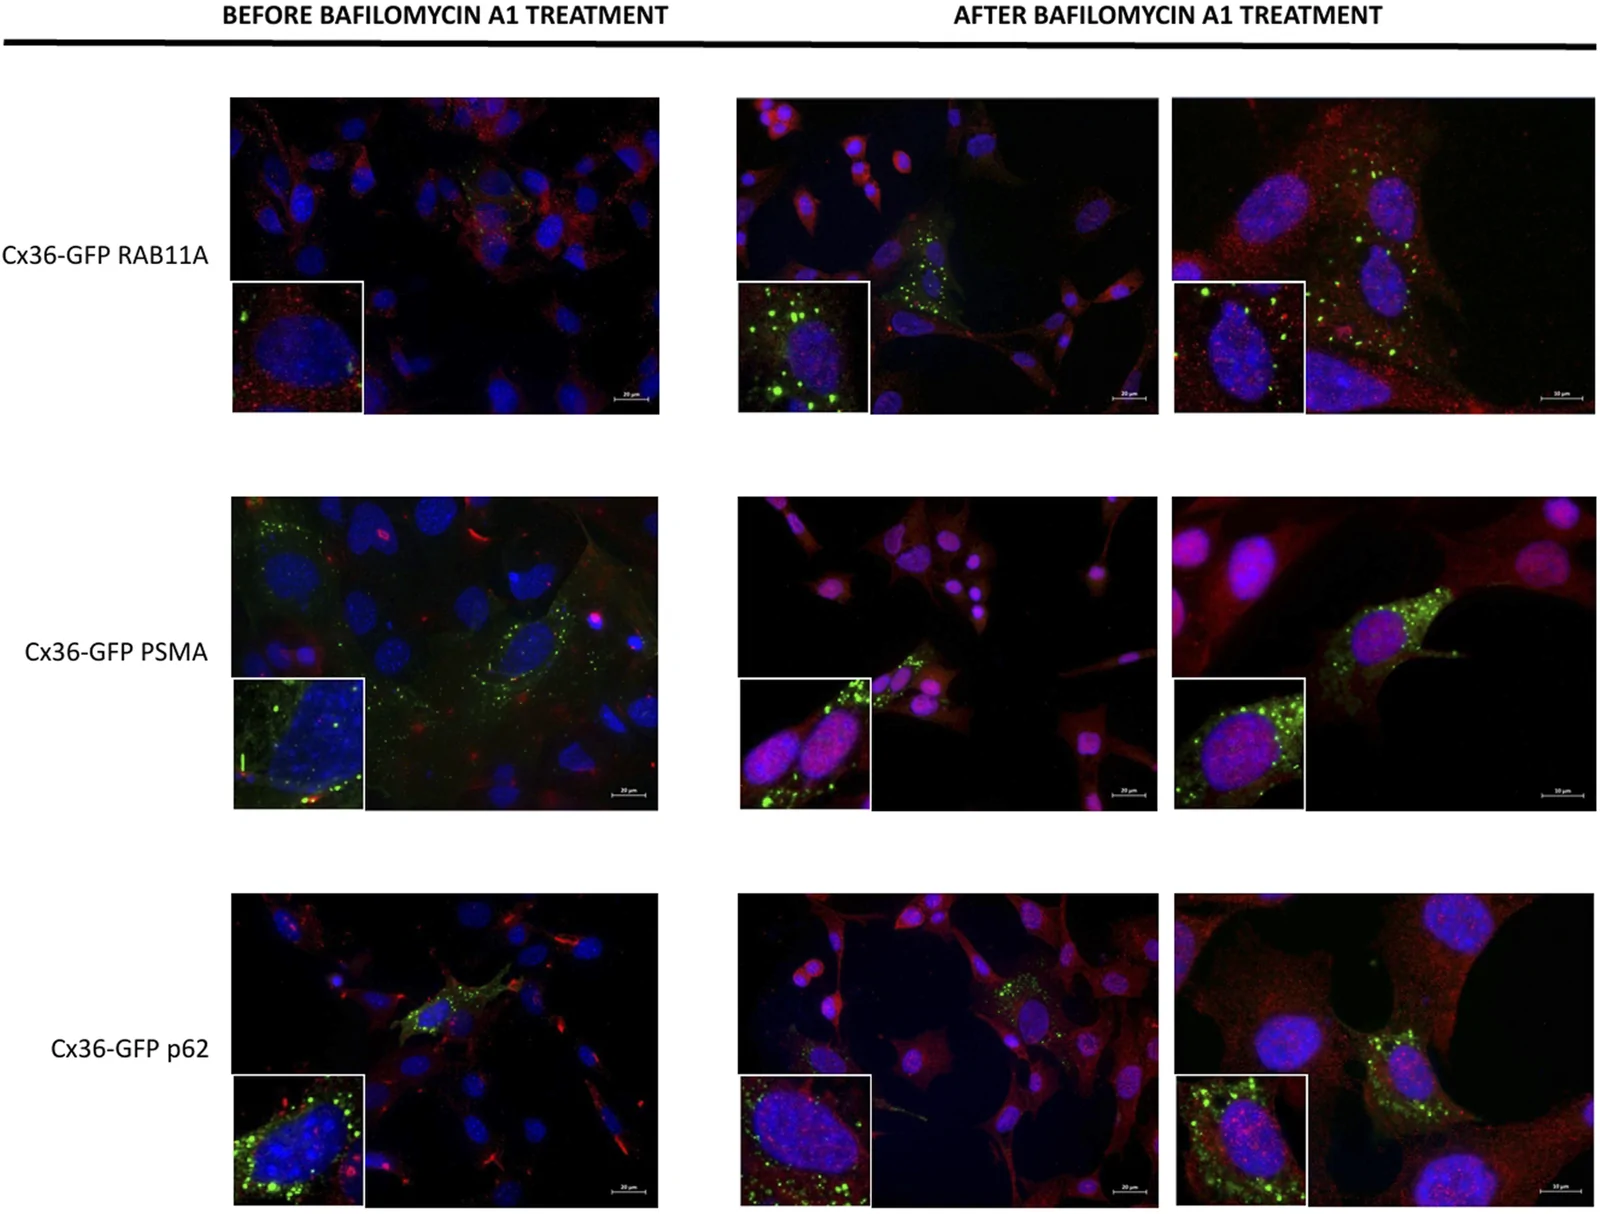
Bafilomycin-induced expression of degradation markers. Green - Cx36-GFP, red - specific marker (RAB11A, PSMA1, p62), blue - DAPI.
